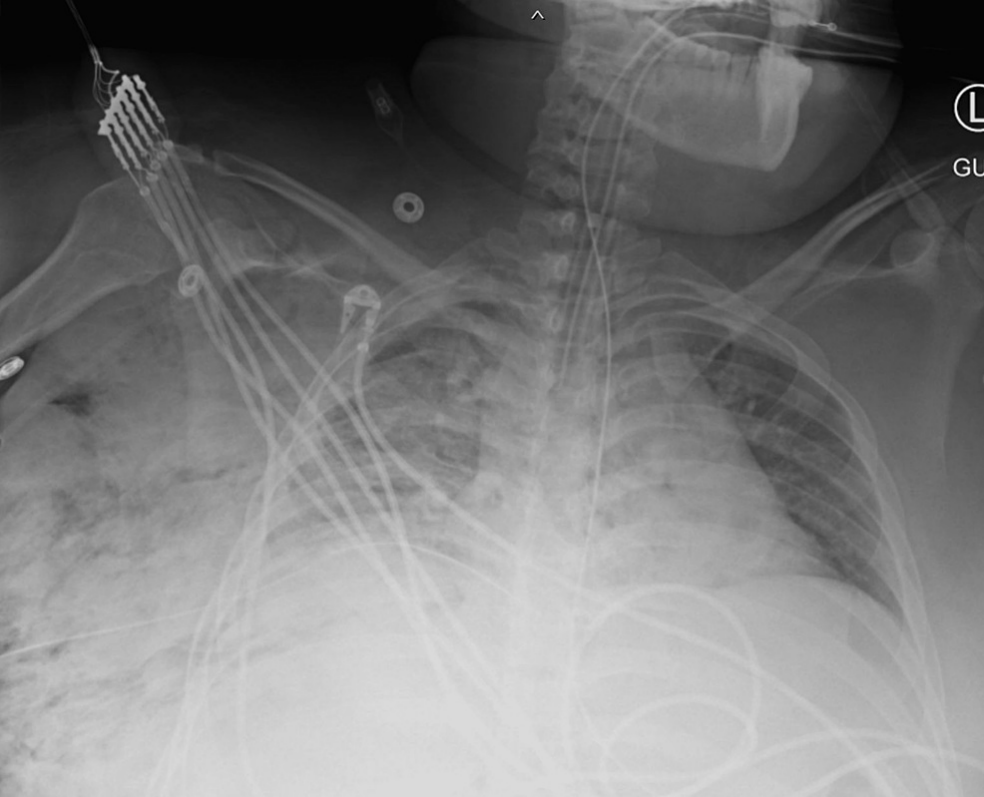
Portable chest X-ray taken 12 hours after tube thoracostomy shows improvement of right-sided subcutaneous emphysema.
